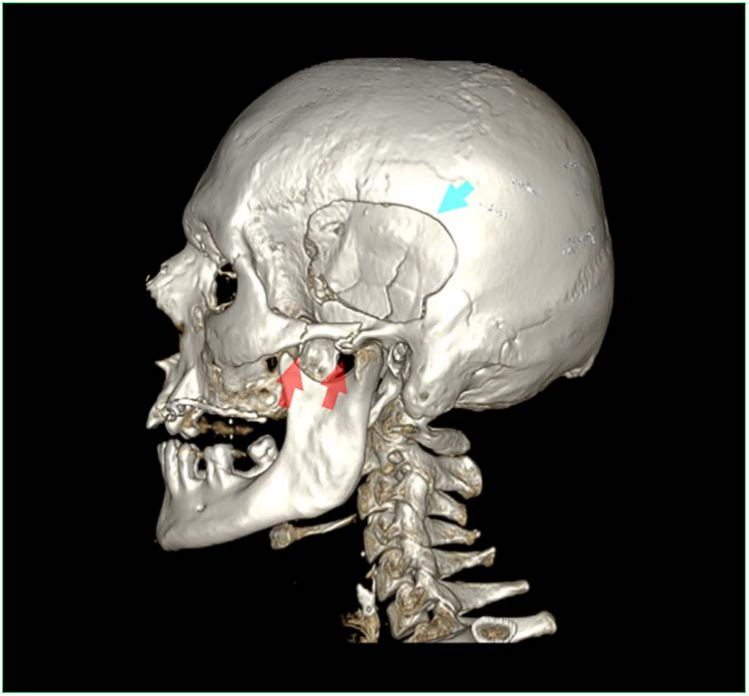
3D reconstruction of the skull and the cervical spine (multidetector computed tomography scan before autopsy), showing the left side of the skull and the cervical spine with visualization of the oval multifragmentary depressed skull fracture in the left temporo-parietal region (blue arrow) and the bifocal fracture of the left zygomatic bone (red arrows).
